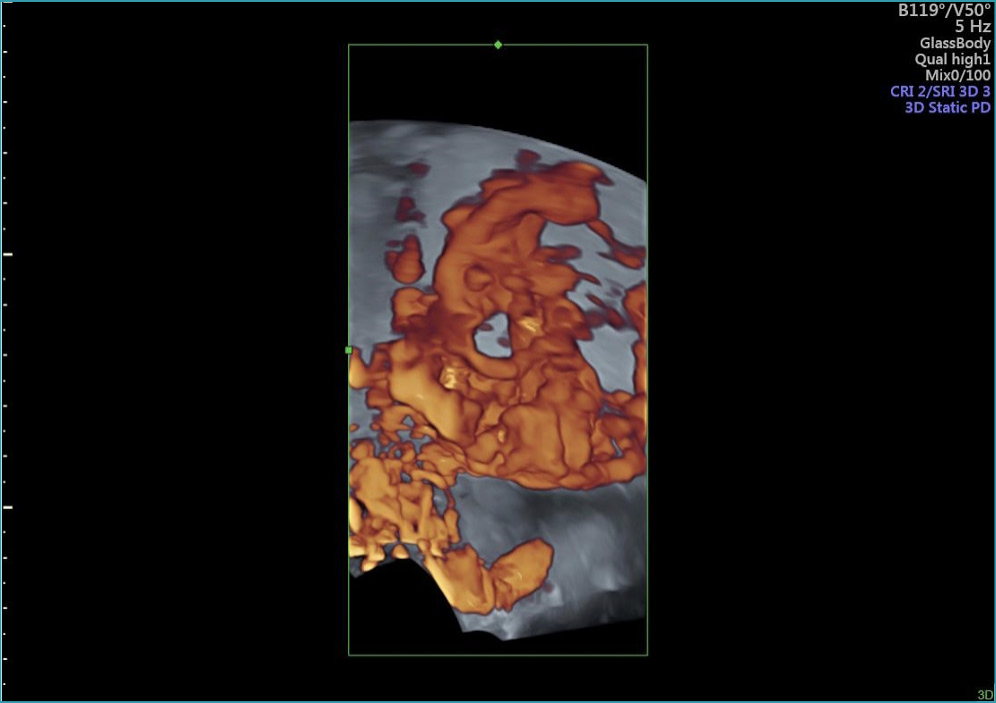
Three-dimensional rendered view of intraplacental hypervascularity associated with power Doppler
